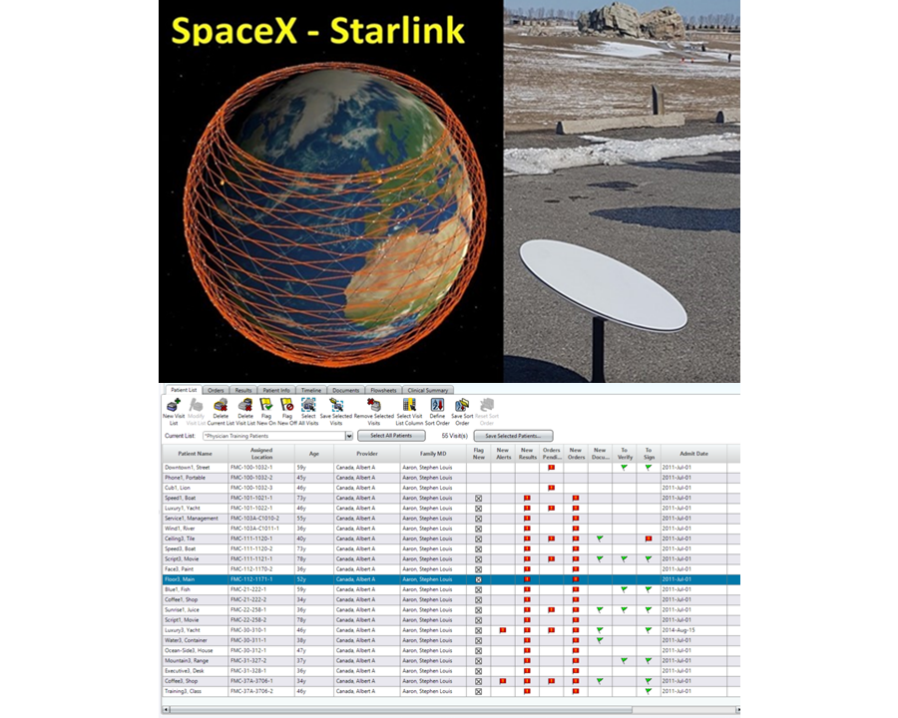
Using the SpaceX Starlink modem (upper panels), the telementored ultrasound (TMUS) team could log into the Allscripts Sunrise Clinical Manager training site by Citrix Workspace, examine patient records, and enter orders (lower panel).
